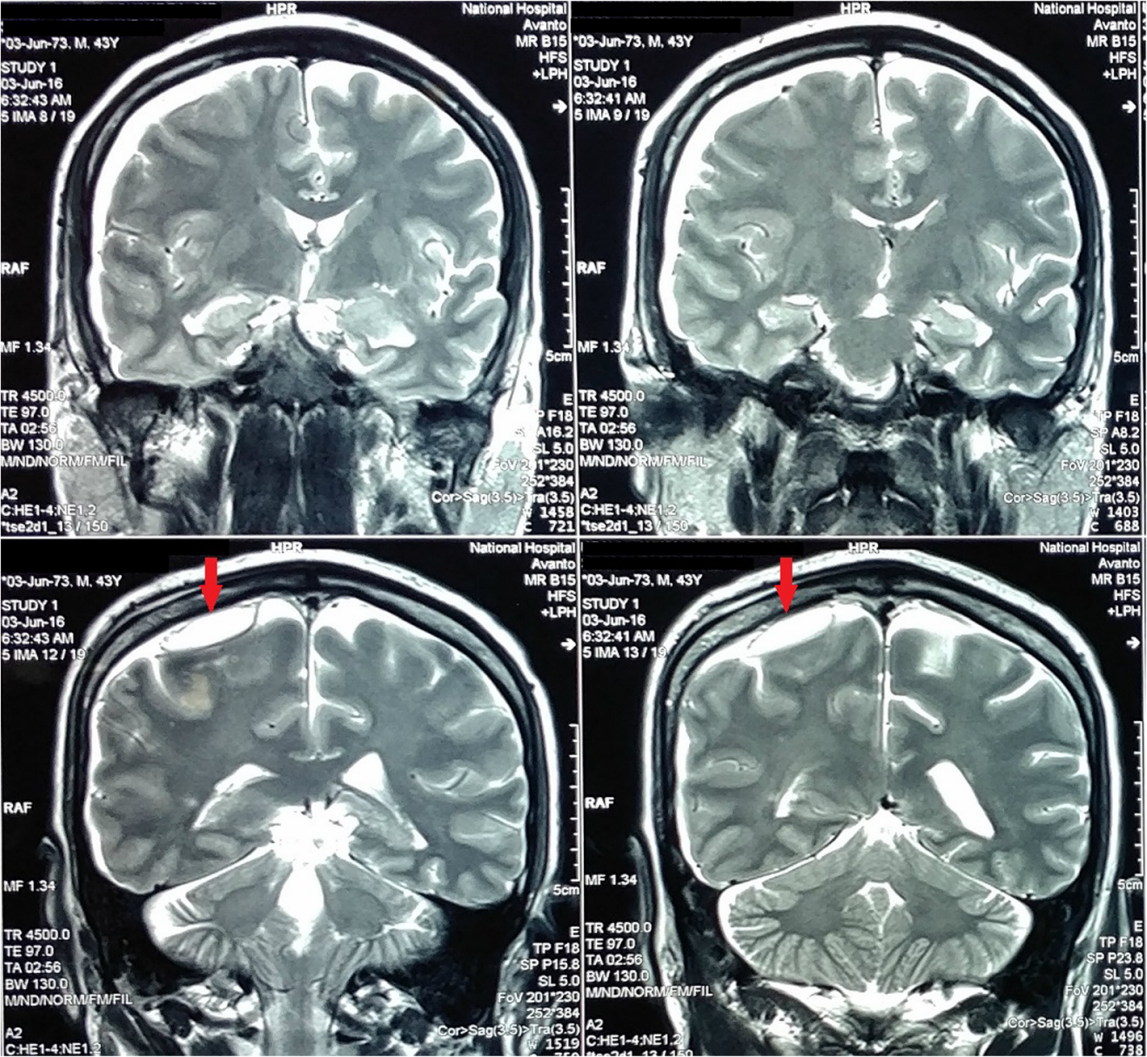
Pre-treatment T2-weighted MRI brain (coronal view) showing a subdural collection in the right fronto-temporo-parietal region with possible abscess formation in the right parietal region
